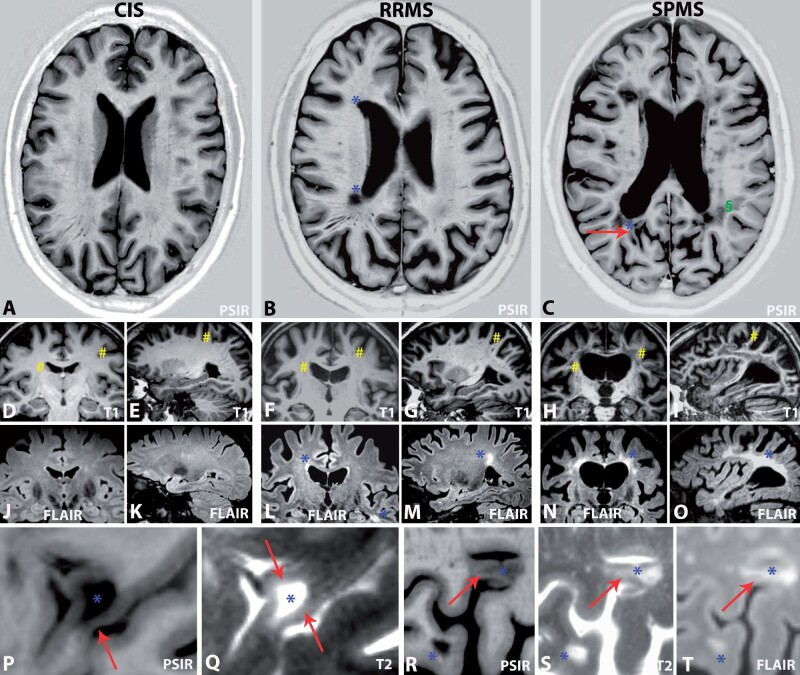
Typical MRI patterns of CIS, RRMS and SPMS. (A, D, E, J and K) A 52-year-old female with CIS (EDSS = 0.0). (A, J and K) No white matter or cortical lesions; (A, D and E) slender ventricles, and no sulcal widening (hash symbol in D and E), brain parenchymal fraction = 0.755. (B, F, G, L and M) A 67-year-old male with RRMS (EDSS = 1.0). Note the multiple sclerosis typical periventricular lesions, suggestive of a perivenous distribution (asterisk in B and M) and typical involvement of the temporal lobes (asterisk in *lower right corner* of L) as well as unspecific periventricular caps (*upper left* asterisk in L), but no cortical lesions. Moderate ventricular and sulcal widening was observed on T_1_-weighted images (hash symbol in F and G), brain parenchymal fraction: 0.727. (C) A 66-year-old female with SPMS (EDSS = 6.5) with leukocortical (arrow in C) and juxtacortical white matter lesions (asterisk and arrow in C) and numerous non-specific deep white matter lesions (section symbol in C). Moderate ventricular and sulcal widening (hash symbol in H and I), brain parenchymal fraction: 0.727. (H, I, N and O) A 53-year-old male with SPMS (EDSS = 6.5) revealed diffuse and widespread signal alterations that affected large portions of the white matter (asterisk in N and O). Severe widening of the inner and outer CSF spaces (hash symbol in H and I), brain parenchymal fraction: 0.658. (P and Q) A 75-year-old female with SPMS (EDSS = 5.5). High magnification images show a leukocortical multiple sclerosis lesion (asterisk in P and Q) with extension of white matter signal alterations into the adjacent cortex (arrow in P and Q) and associated cortical atrophy (arrow in P). (R–T) A 57-year-old female with RRMS (EDSS = 2.5). Leukocortical lesions (*top* asterisk and arrows in R–T) are distinct from juxtacortical lesions (*bottom* asterisk in R–T), which (based on the available sequences) abut the cortical surface and show no morphological evidence for cortical involvement. Supplementary Table 2 contains all clinical and quantitative MRI biomarker details.
